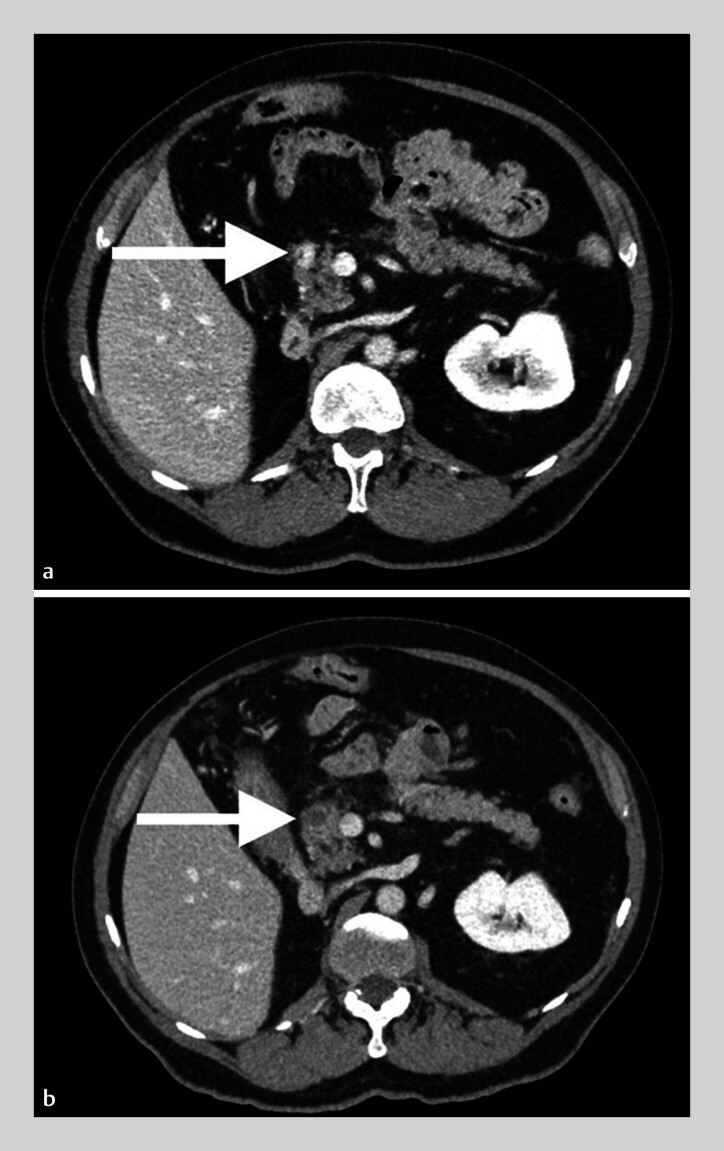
Abdominal CT scan of Patient 2
